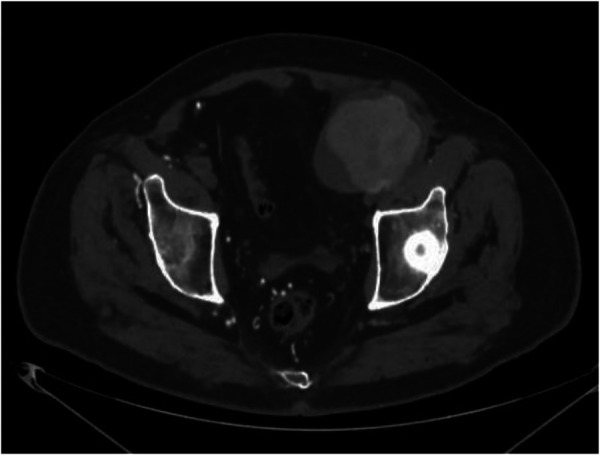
Computed Tomography-angiography axial scan: voluminous pseudoaneurysm at the level of the left branch of the bifemoral aorto bypass.
